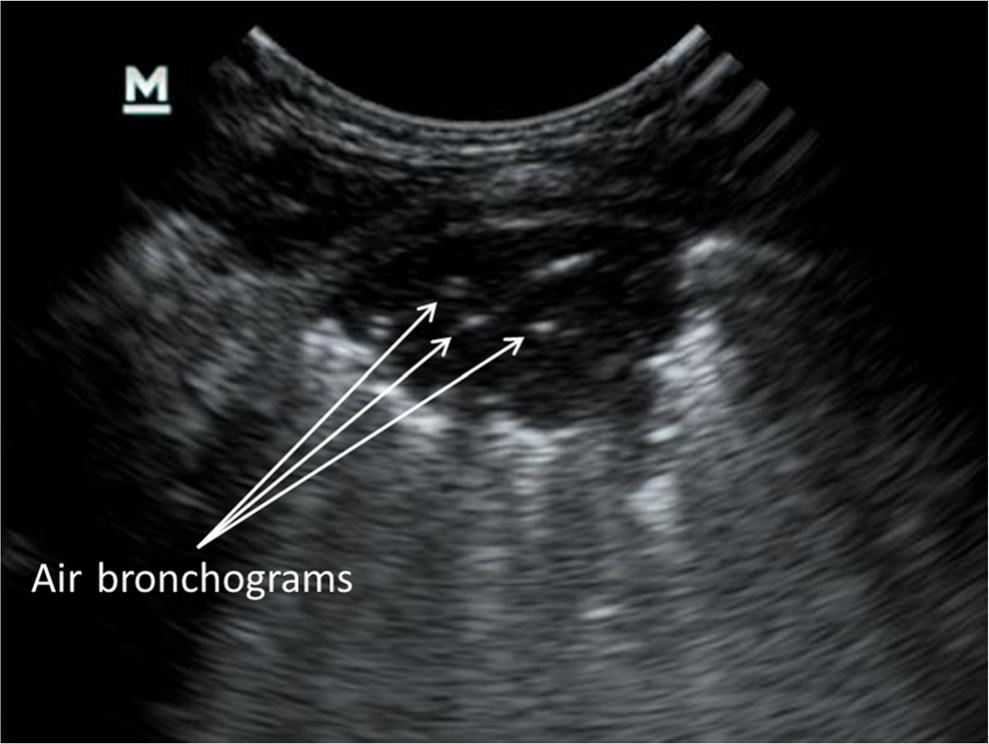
An ultrasound image from the right posterior upper lung zone in a 3-month-old girl hospitalised with pneumonia shows a wedge-shaped hypoechoic area of subpleural consolidation. Associated features that can be seen are air bronchograms represented by punctate hyperechoic specs within the lesion, a hypoechoic pleural line over the lesion and multiple B-lines that arise from the deep edge of the consolidation rather than from the pleura
